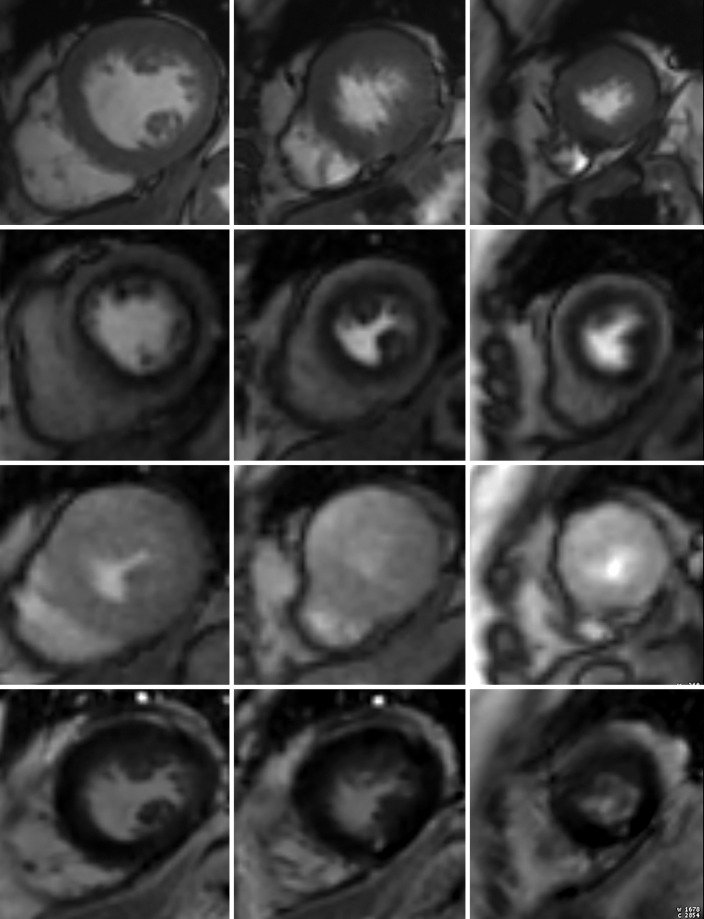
Stress perfusion imaging in a 53-year-old woman with chest pain on exertion and at rest. (a) From left to right in the top row, basal, mid, and apical short-axis cine MR images of the LV show normal global and regional left ventricular systolic function (LV ejection fraction, 61%). In the second row, adenosine stress perfusion images of the same LV locations as in the top row show severe subendocardial perfusion defects involving all myocardial segments. In the third row, the rest perfusion images of the same LV locations are normal. In the fourth row, the LGE images of the same short-axis LV locations show a small focus of enhancement that may represent a microinfarction or an embolic infarction in the mid to apical inferior wall. (b, c) Left anterior oblique (LAO) (b) and LAO caudal (c) invasive coronary angiographic images show severe proximal and distal right coronary artery stenoses on the LAO view (arrows in b), as well as severe stenosis of the distal left main coronary artery on the LAO caudal view (arrow in c).
